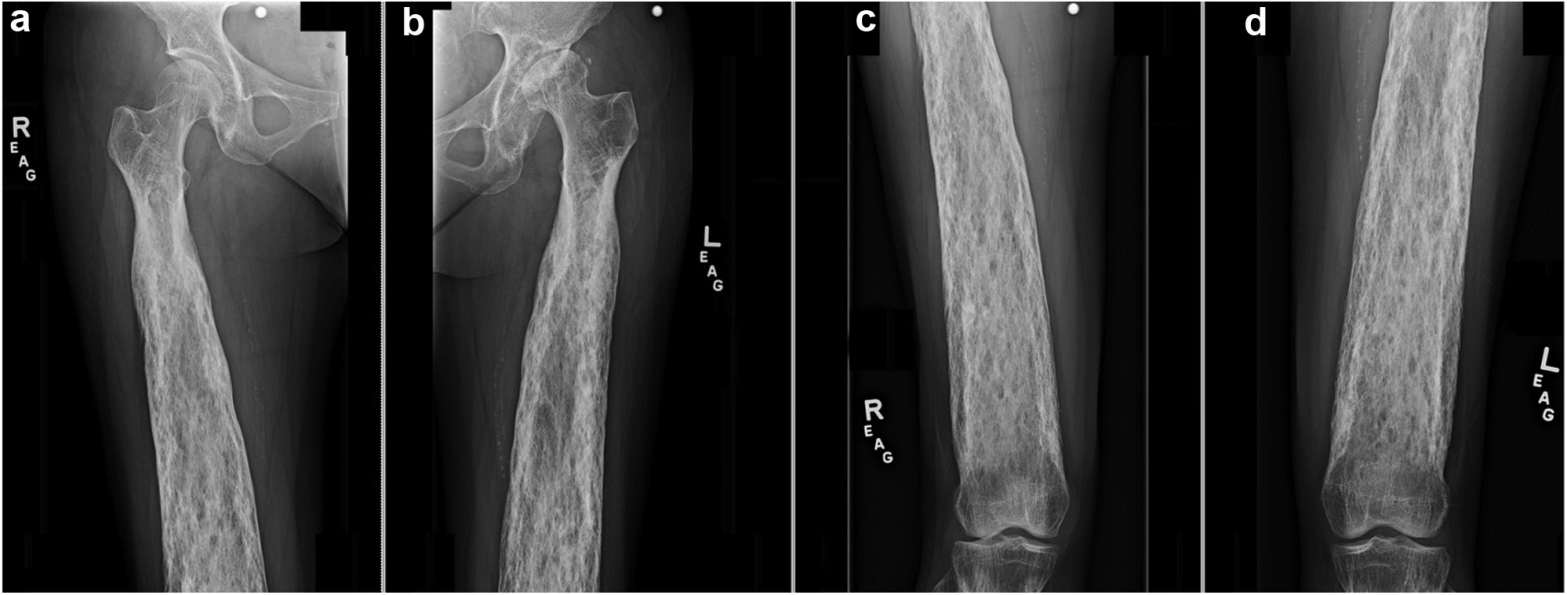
Preoperative anteroposterior radiographs of the right (a, c) and left (b, d) femurs demonstrating symmetric, bilateral cortical thickening and broadening of the diaphysis.
